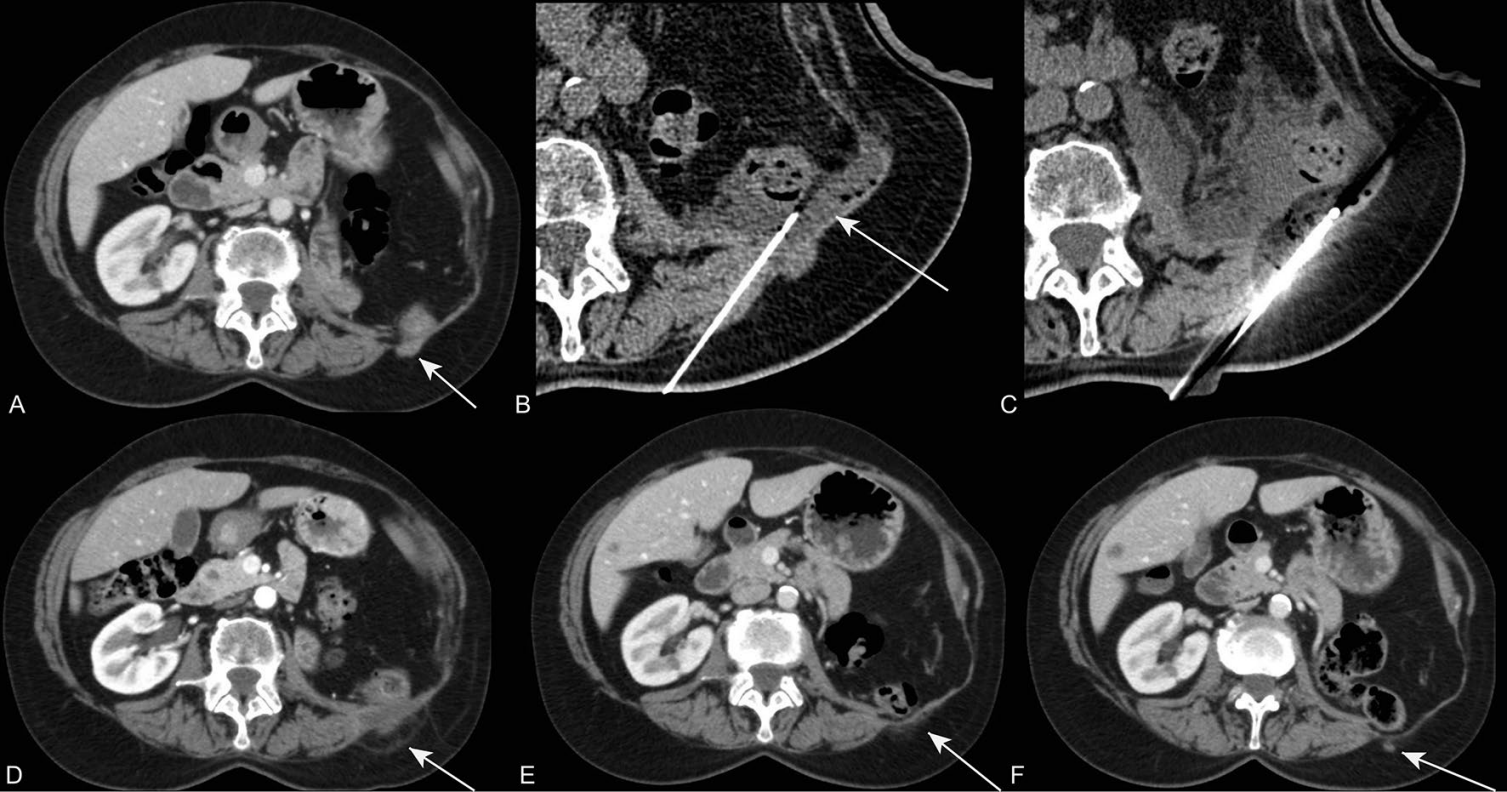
A 76-year-old female patient with a non-visceral metastases of RCC in the abdominal wall of 22 mm (A) after nephrectomy, which was previously treated with SBRT and twice with radiofrequency ablation. Hydrodissection (B) was performed for bowel proximity and one needle was used during cryoablation (C). Follow-up CT shows complete ablation after one month (D), and persistent local control after 8 months (E). Local tumor progression was detected after 14 months (F) which was left untreated and closely monitored with watchful waiting
